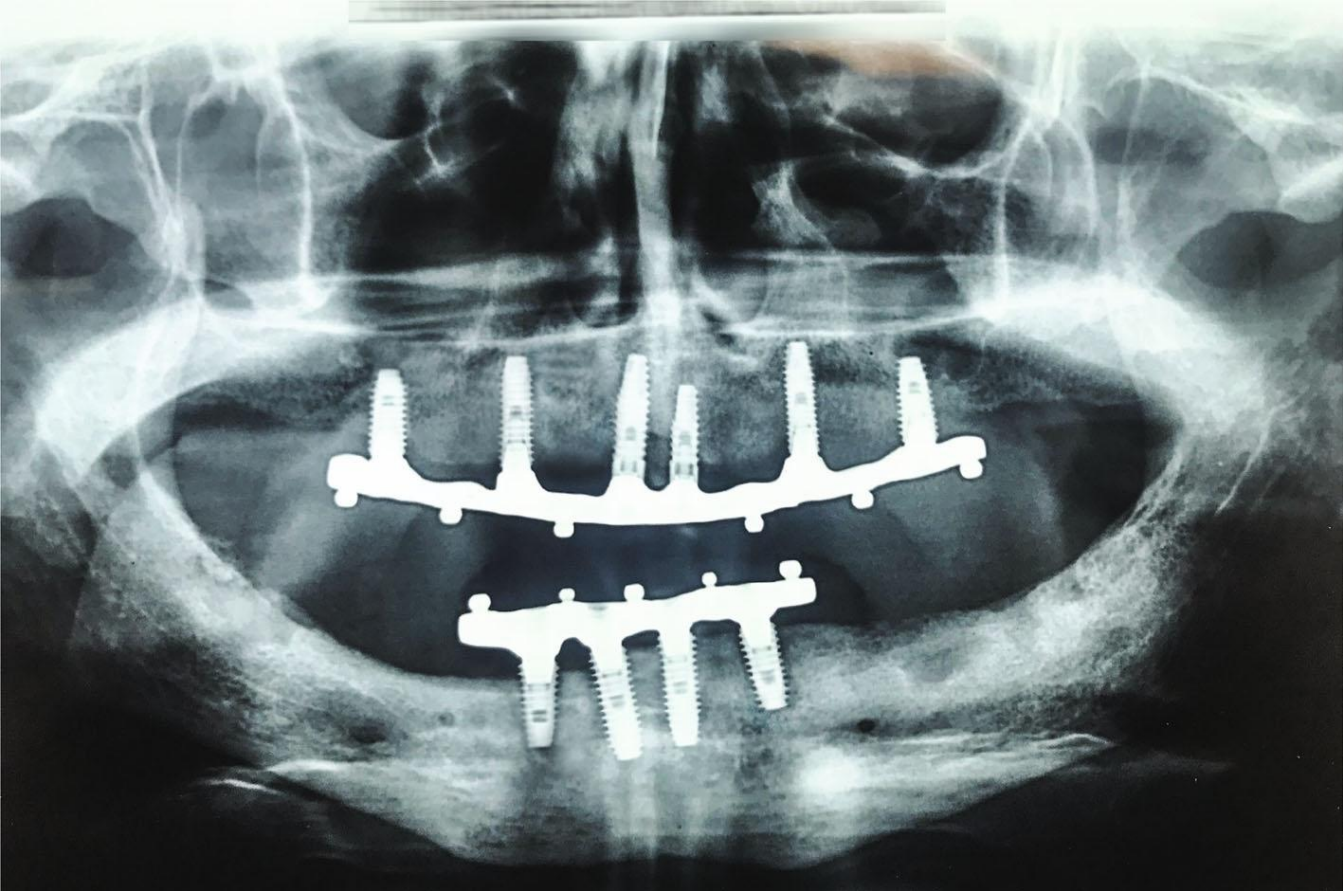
Follow-up panoramic radiograph 10 months after loading.
